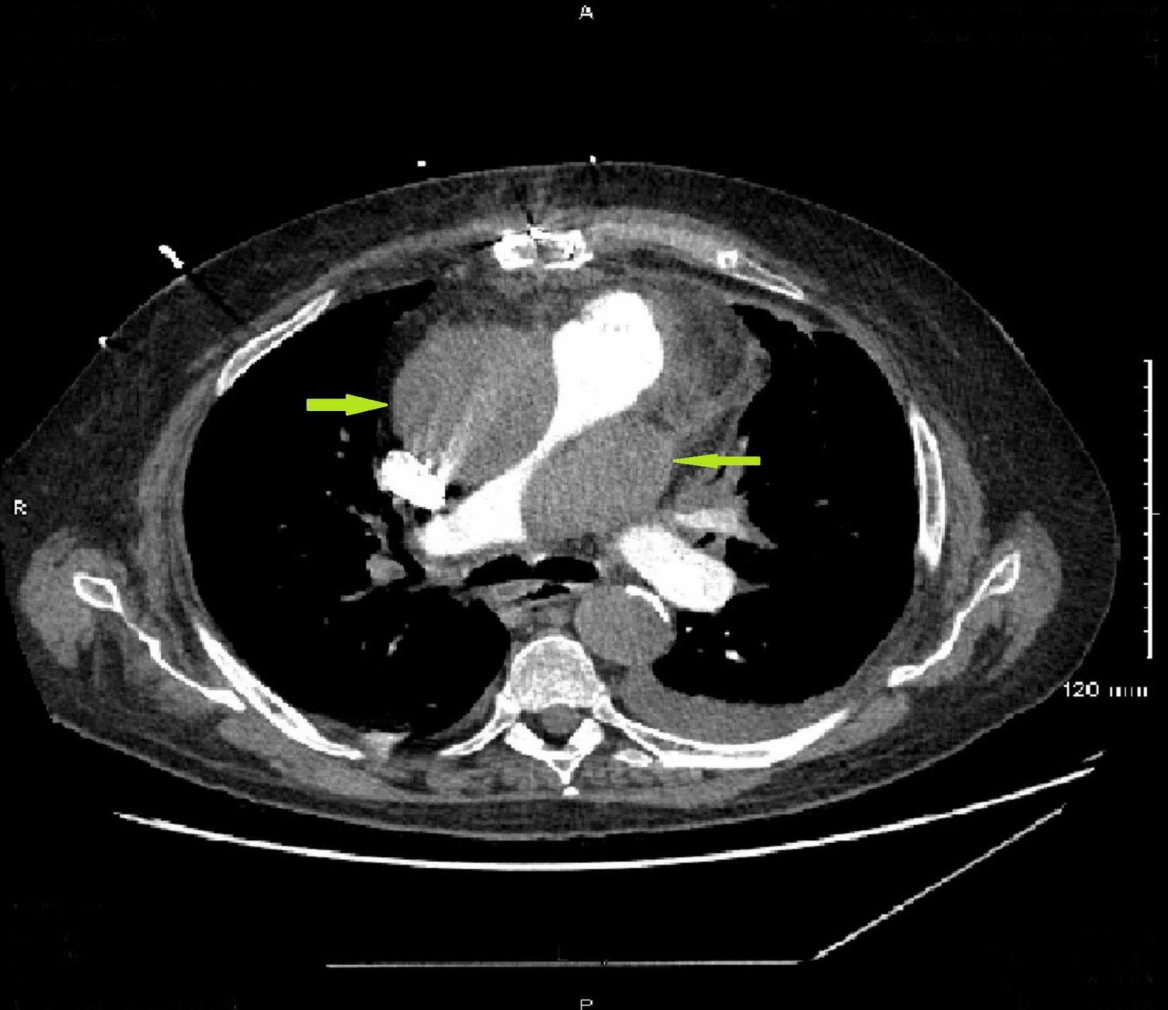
Computed tomography pulmonary angiogram (CTPA) CTPA without evidence of pulmonary embolism, however, shows a marked mass effect on the pulmonary trunk and right pulmonary artery (green arrows).
